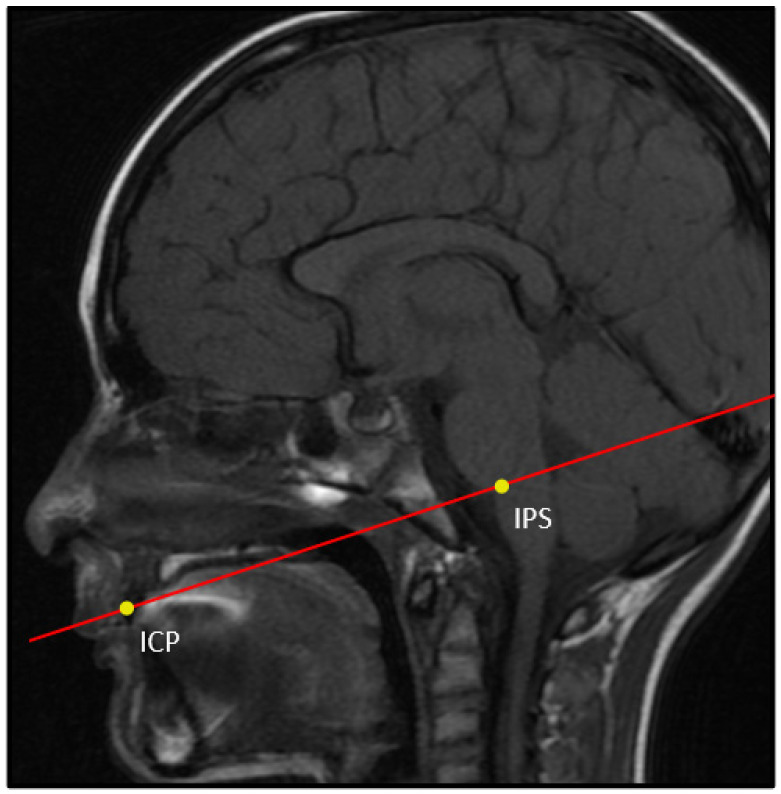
Midsagittal slice of the initial sagittal MRI used to specify the oblique axial scan through the plane of the inferior pontine sulcus and incisive occlusion point. IPS: inferior pontine sulcus (groove between the pons and medulla oblongata); ICP: incisive occlusion point (point of contact between the right and left upper central incisors).
